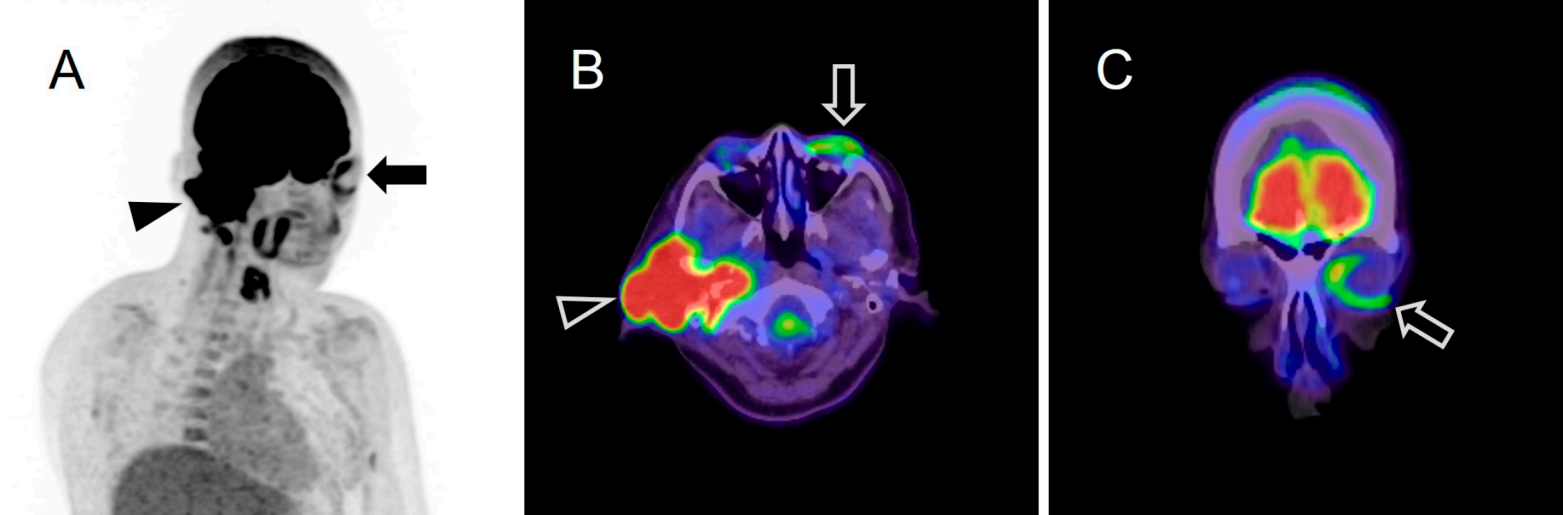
Positron emission tomography/computed tomography showing intense ^18^F-FDG uptake associated with right external auditory canal cancer (A, B: arrow heads) and increased ^18^F-FDG uptake (maximum standardized uptake value: 3.08) in the left orbicularis oculi muscle on the unaffected side (A, B, C: arrows). ^18^F-FDG: ^18^F-fluorodeoxyglucose.
